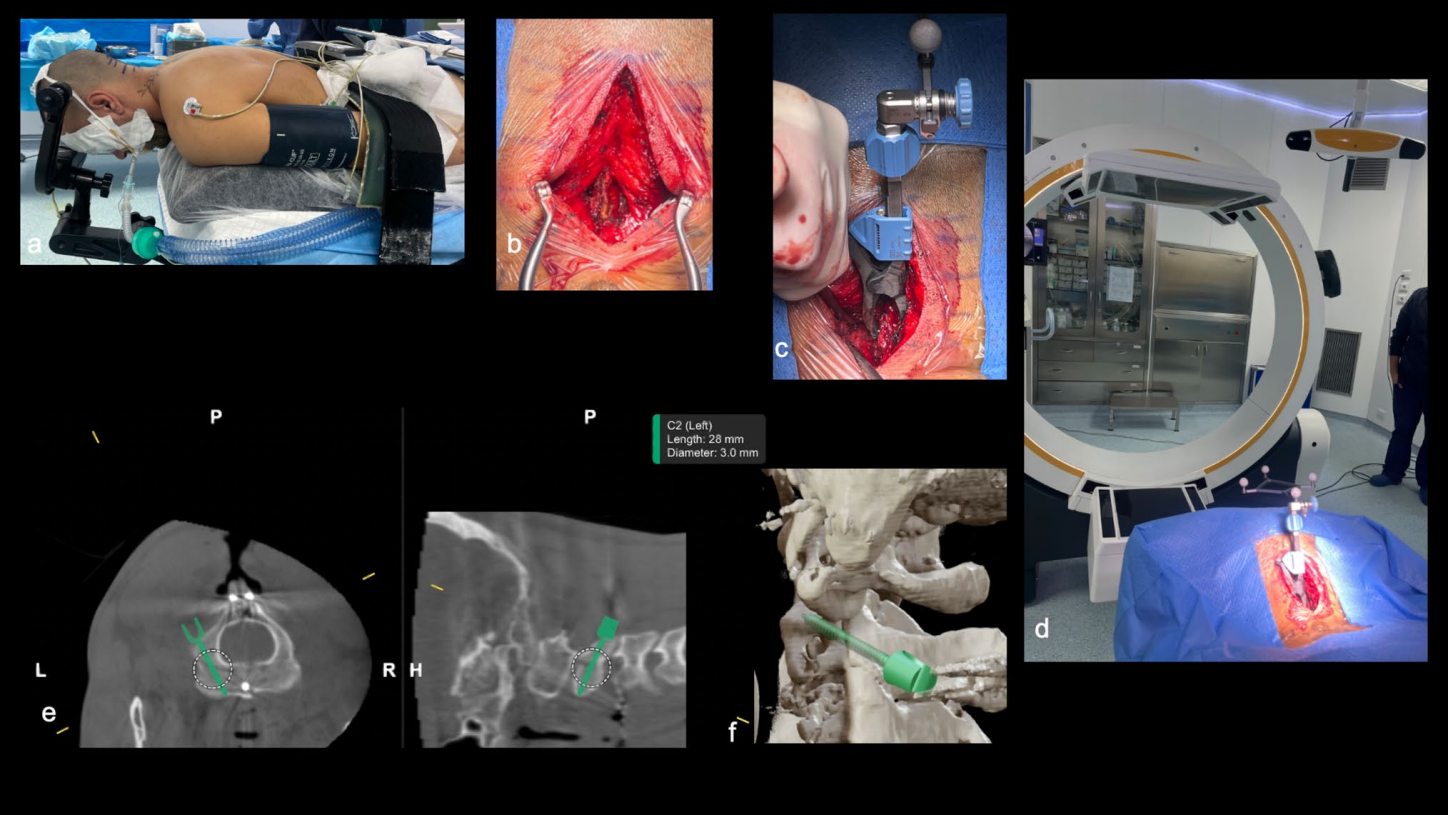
a Patient positioning in the operating room in a prone position using a three-point radiolucent head holder. b Midline posterior cervical skin incision, exposure of the C2 spinous process, laminae, and articular processes, followed by fixation of a navigation reference to the C2 spinous process (c). d Operating room setup with the LoopX imaging system and navigation camera (Brainlab) aligned to the reference secured to the patient. e Preoperative virtual planning on the Brainlab neuronavigation workstation, using the LoopX CT scan to define the trajectory, entry point, and dimensions of the screws for the unilateral fracture of the left pedicle (with CT images maintaining natural left–right orientation); 3D reconstruction view (f)
